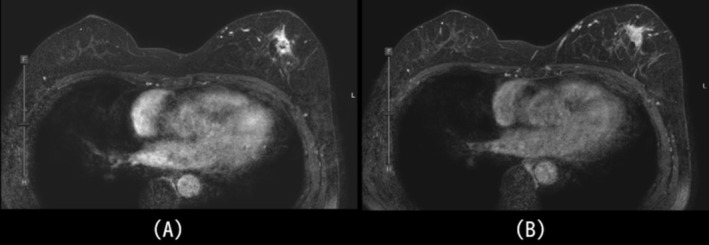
Dynamic contrast‐enhanced MRI images of the breast. (A) Early‐phase image shows a 1.3‐cm irregular mass with rapid enhancement. (B) Delayed‐phase image demonstrates persistent enhancement with irregular margins.
